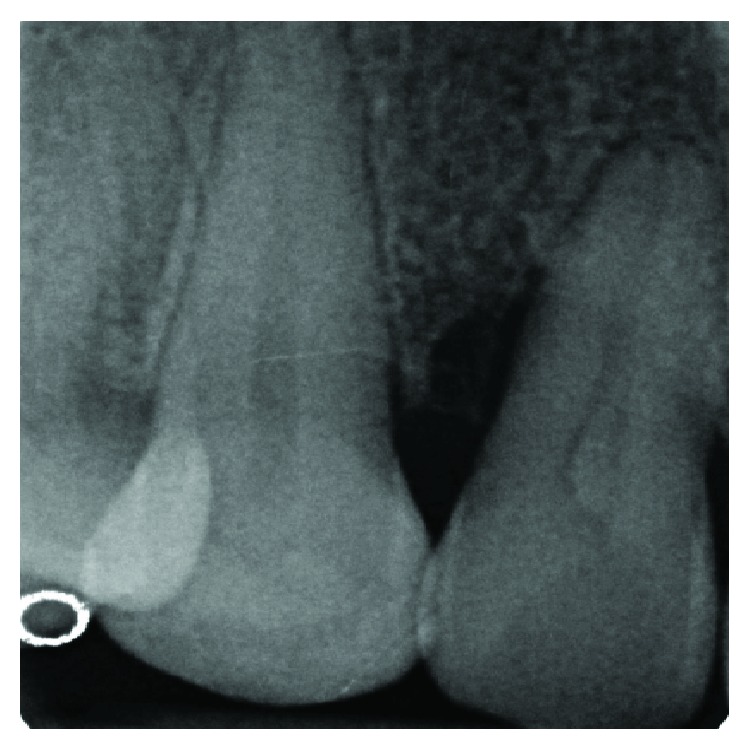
Radiographic examination: no bone resorption is visible in correspondence of the involved tooth.
